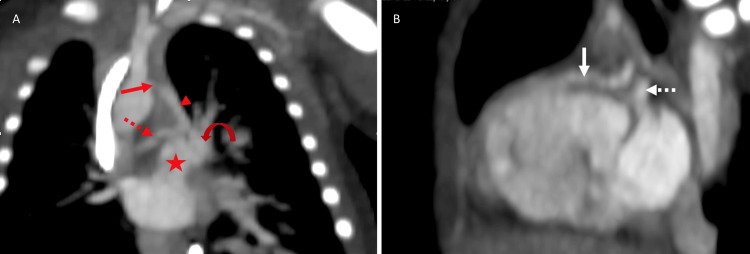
Cardiac CT angiography in coronal (A) and sagittal (B) plane. (A) The coronal image shows hypoplasia of the pulmonary trunk (star) and its left (curved arrow) and right (red dashed arrow) branches. Additionally, there is persistence of the ductus arteriosus (red arrowhead) with narrowing towards the aortic arch (red arrow), which reaches the right pulmonary artery branch. (B) The sagittal image depicts the outflow tract of the left ventricle (white arrow) and the presence of an aortopulmonary collateral (white dashed arrow).
